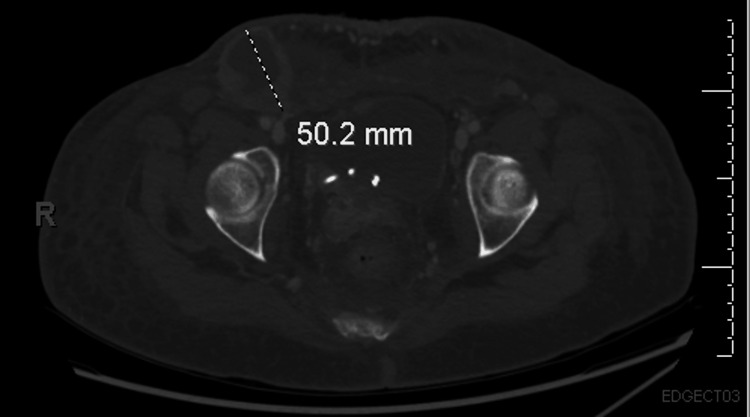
CT scan of the pelvis with contrast revealing a hypovascular lesion measuring 50.2 mm in diameter, characterized by thickened enhancing walls within the right inguinal region, resembling a large necrotic lymph node
